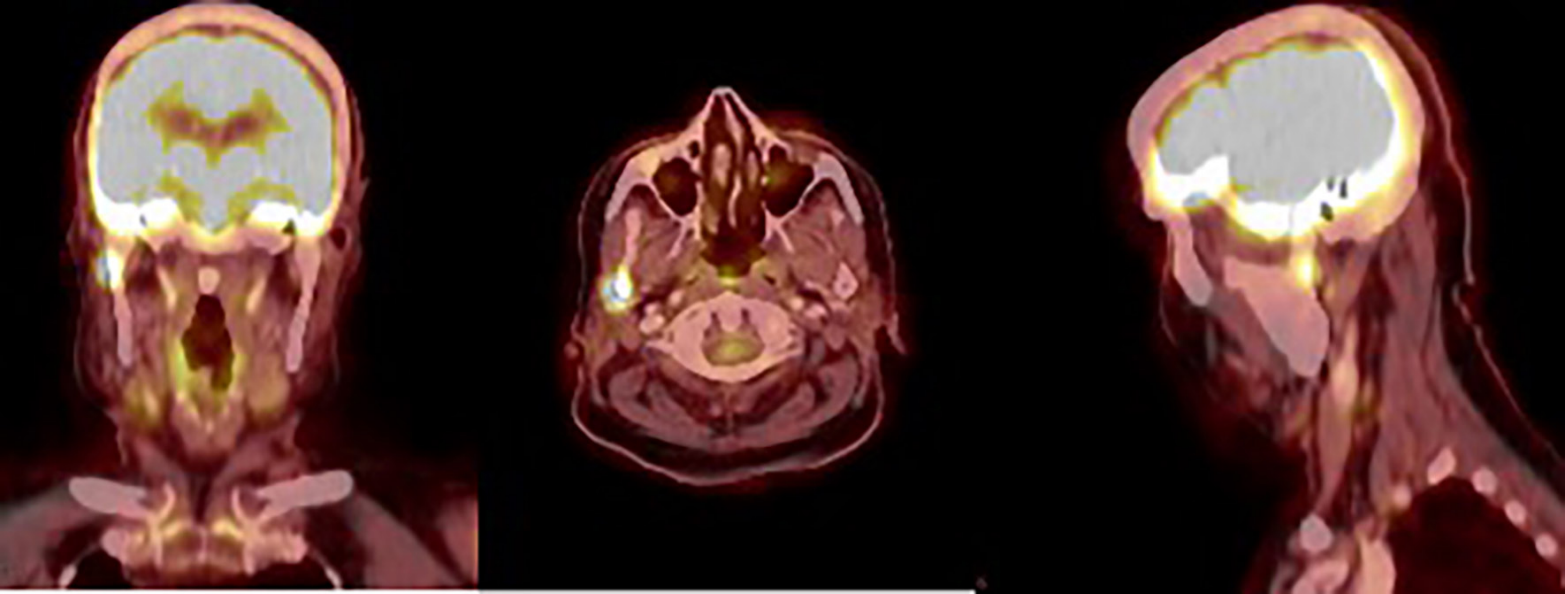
PET-CT 18F-FDG showing focal uptake in the right mandibular condyle.
